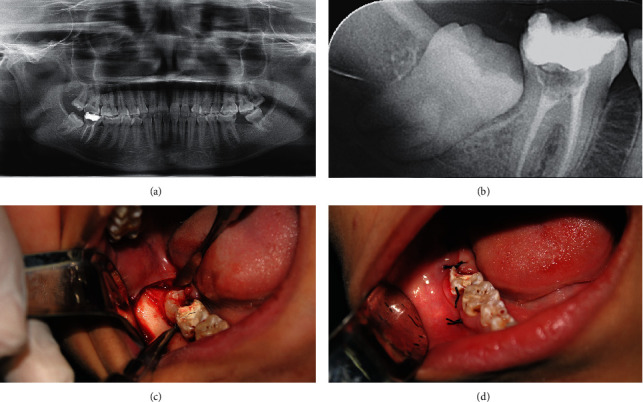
Surgical extraction of mandibular right 3^rd^ molar for Case 2. Presurgical evaluation included panoramic (a) and periapical (b) radiographic assessment in addition to clinical examination. The procedure was started by a pyramidal flap incision followed by mucoperiosteal flab elevation (c). Next, bone removal to expose the impacted molar was completed as needed followed by guttering buccally and distally. The site was sutured with simple interrupted sutures using 3-0 silk and hemostasis achieved (d).
